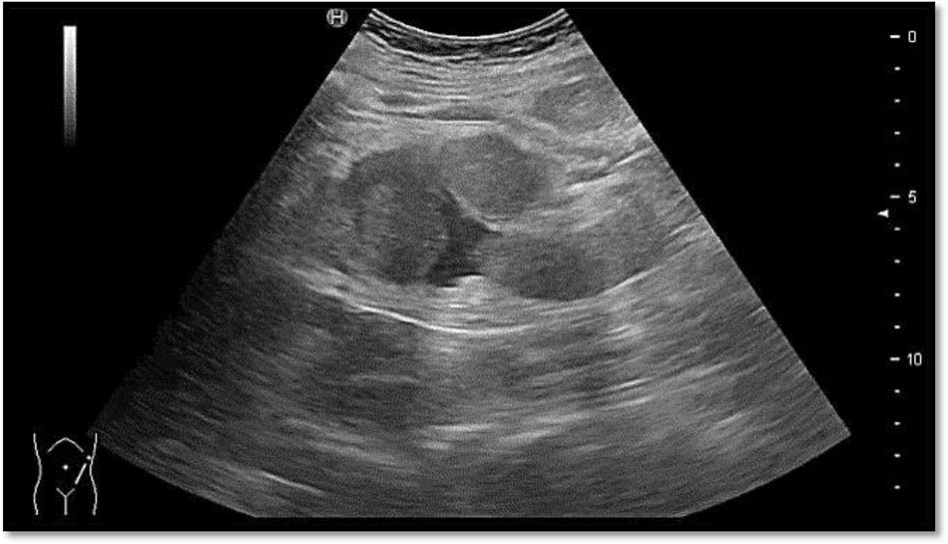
Abdominal sonography in the emergency department with free fluid on the left hemiabdomen.
